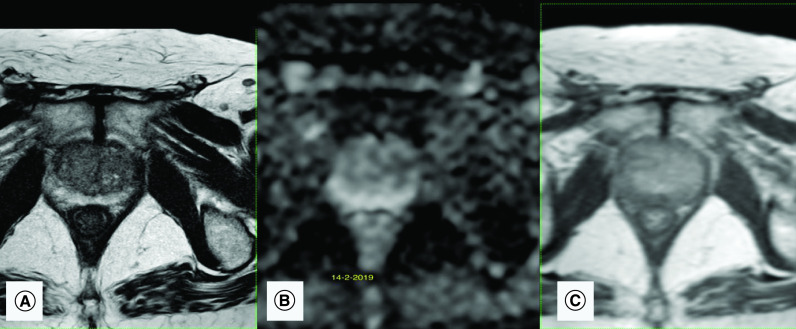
Multiparametric prostatic MRI before intravesical bacillus Calmette–Guérin therapy. (A) Axial T2-weighted image shows peripheral symmetrical hypointense linear lesions corresponding to sequelae of chronic prostatitis. (B) Apparent diffusion coefficient map of the diffusion-weighted image shows no corresponding diffusion restriction in peripheral zones. (C) Dynamic contrast enhancement shows only mild enhancement mainly in the left peripheral zone. These findings suggest sequelae of chronic prostatitis and a PI-RADS score of 2.
